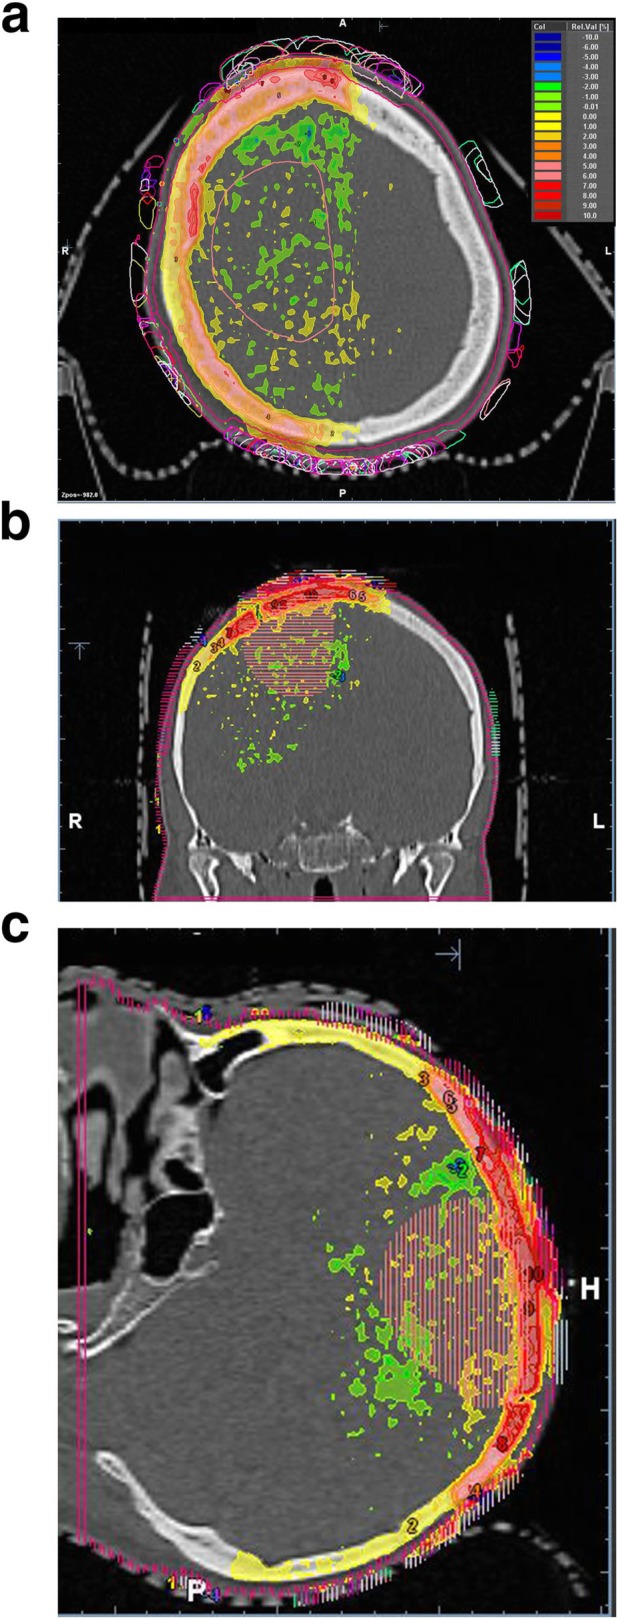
(a-c) Highlighting dose difference plots of accumulated dose distributions with and without electrodes (a-c): Delineated clinical target volume (right frontoparietal), 2mm shell contour and TTF array structures over the whole series (a) right: axial computed tomography; (b) middle: aligned, coronar reconstruction; (c) left: sagittal reconstruction. Dose differences are expressed as percentages of the prescribed dose
